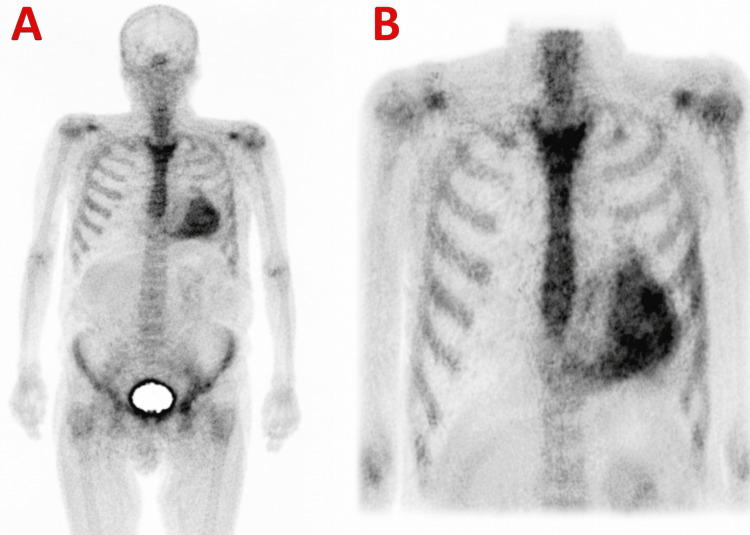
Bone scintigraphy confirming ATTR-CM 99mTc-HMDP bone scintigraphy demonstrating intense diffuse myocardial tracer uptake exceeding rib uptake, corresponding to a Perugini grade 3 pattern, is highly suggestive of ATTR-CM. (A) Whole-body anterior planar image showing prominent cardiac radiotracer uptake. (B) Magnified thoracic view demonstrating marked myocardial uptake with relative attenuation of rib uptake, confirming the diagnosis of ATTR-CM in the absence of a monoclonal gammopathy. ATTR-CM: transthyretin cardiac amyloidosis, 99mTc-HMDP: technetium-99m hydroxymethylene diphosphonate
